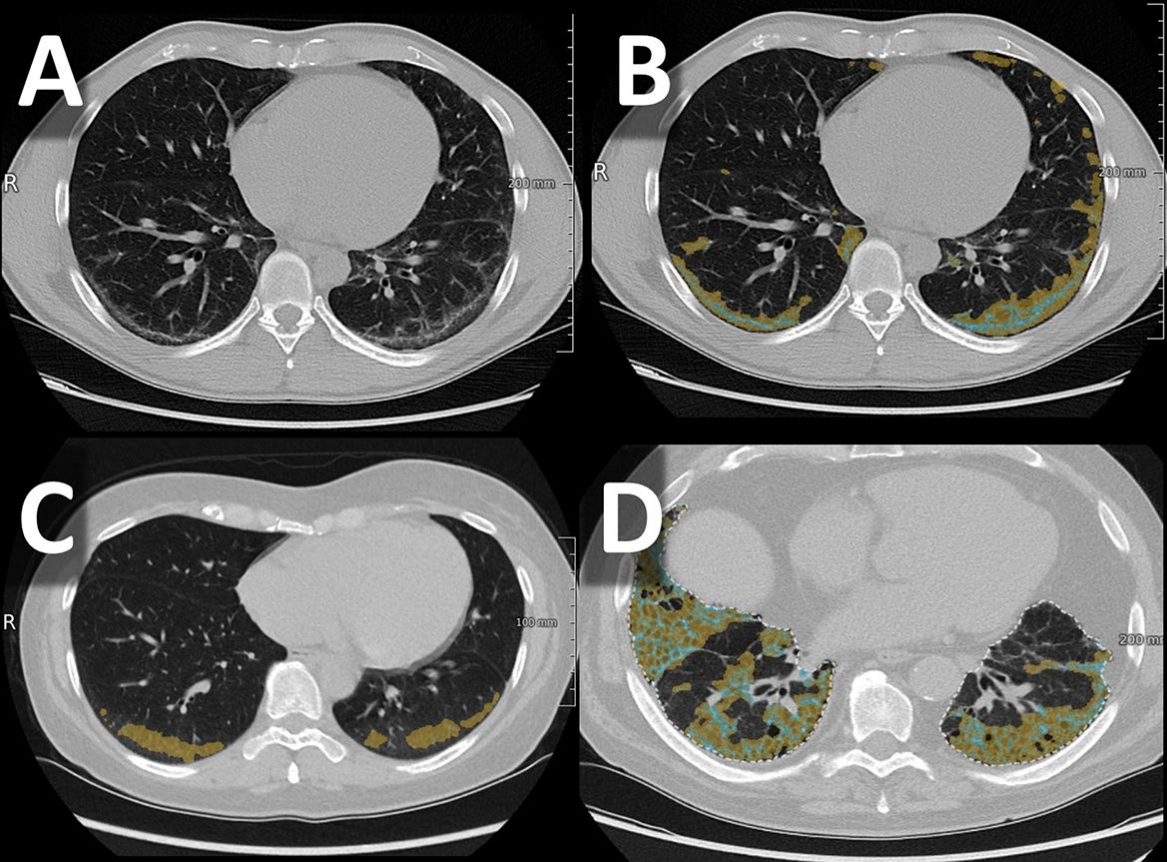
Different high-resolution computed tomography (HRCT) patterns with AI-based segmentation of pulmonary HRCT using SATORI; A – Non-specific interstitial pneumonia without AI-based segmentation; B – Non-specific interstitial pneumonia; C – Ground glass opacities; D – Usual interstitial pneumonia
